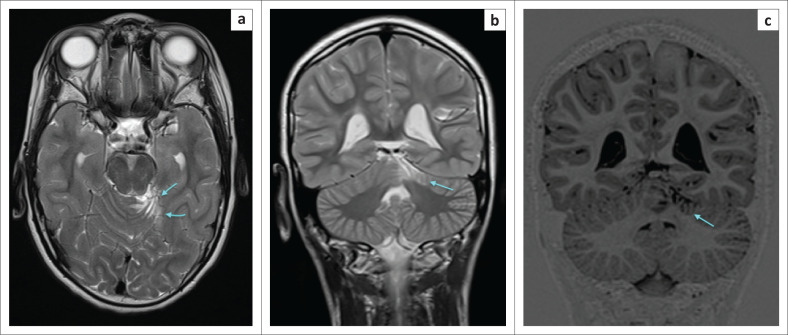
A 9-year-old male child who suffered grade 3 hypoxic ischemic brain injury with Apgar scores of zero at 1 min and 7 at 5 min. (a) An axial T2-weighted, (b) a coronal T2-weighted and (c) a coronal inversion recovery (IR) sequence image demonstrating the left superior cerebellar cortex injury (cyan blue arrows) with localised atrophy involving the left half of the quadrangular lobule. This is an important review area, as in this case, the injury was omitted by the first reporter.
